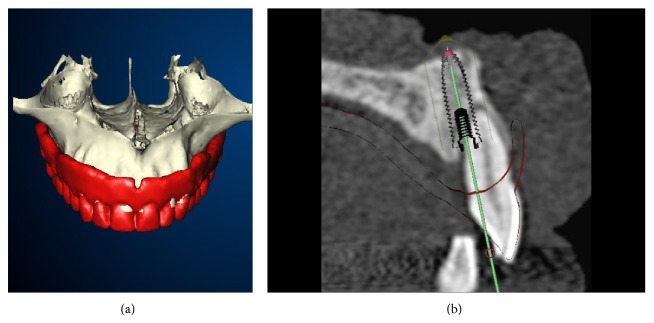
(a) Even if this template has never been worn by the patient, it can be merged to the patient CT data because of the radiographic markers. (b) Respect of the prosthetic project is easier with the computer-aided technology than in conventional hands-free procedure for immediate implants.
